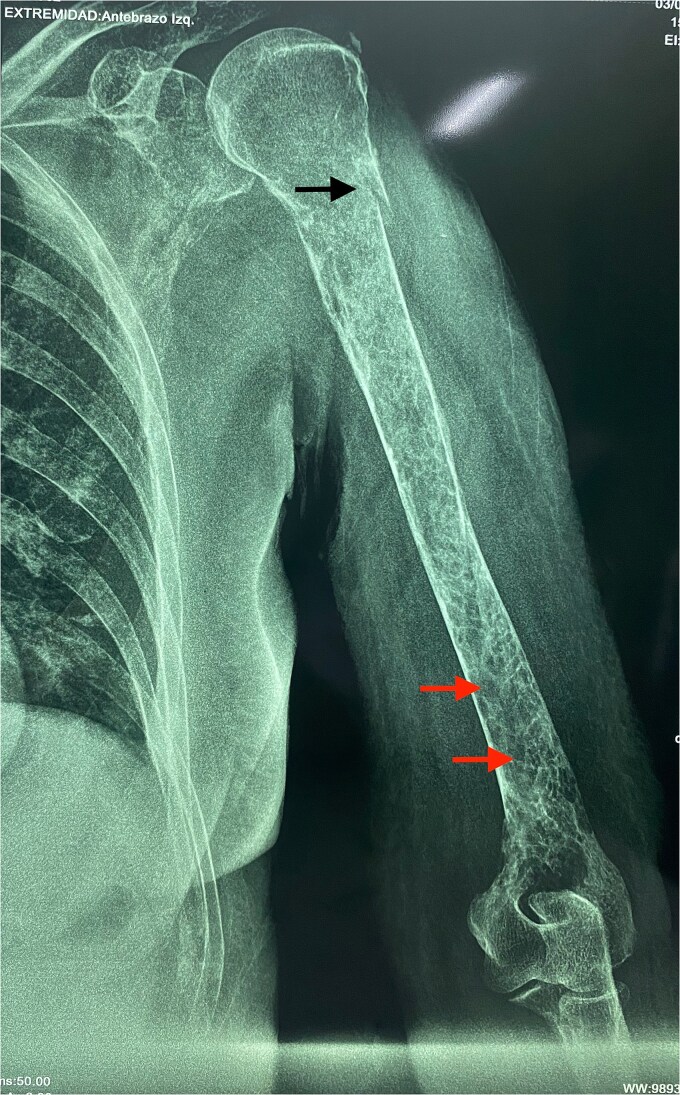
Radiograph of the left humerus in a 64-year-old woman with oligosecretory multiple myeloma, showing a fracture at the surgical neck (black arrow) and lytic lesions in the distal third of the bone (red arrows).
